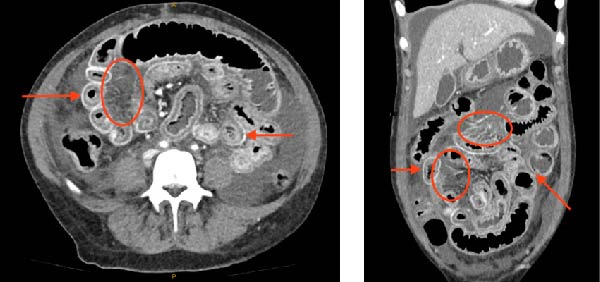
Portal phase CT abdomen of the patient acquired on presentation to the emergency department—as pictured, this demonstrated marked circumferential bowel wall thickening throughout the entire length of the small bowel and proximal colon (‘target sign’, demonstrated by red arrow). Engorgement of the mesenteric vessels (‘combs sign’) with attenuation of the mesenteric fat was also commented on (demonstrated by red circle), along with large volume free fluid. These signs are helpful in the identification of SLE enteritis [2].
